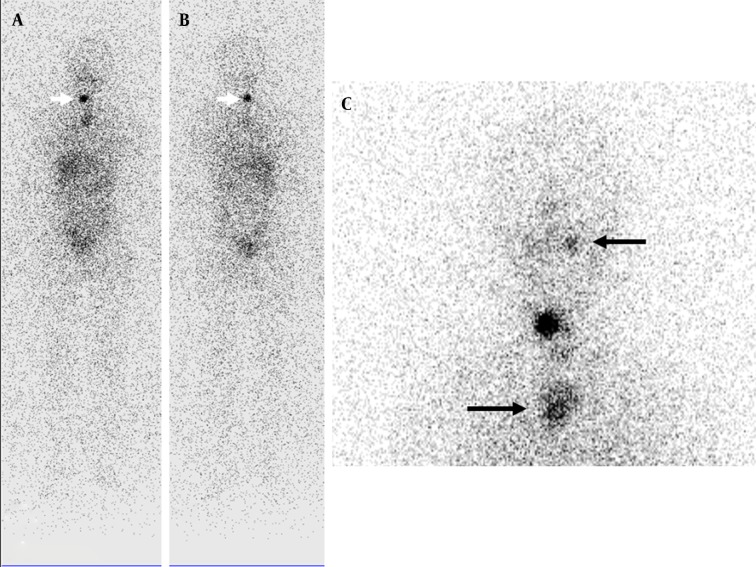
Anterior and Posterior Whole-body Scan Images and Neck Static Image Demonstrating Radioiodine Accumulation in Thyroid Bed . Less intense activities in left maxillary and upper thoracic regions were seen that belonged to dental inflamation and thymic activity, respectively (black arrows). Thoracic computed tomography scan was performed after the radioiodine scan showed a heterogeneous mass in the mediastinum, suggestive of thymus.
